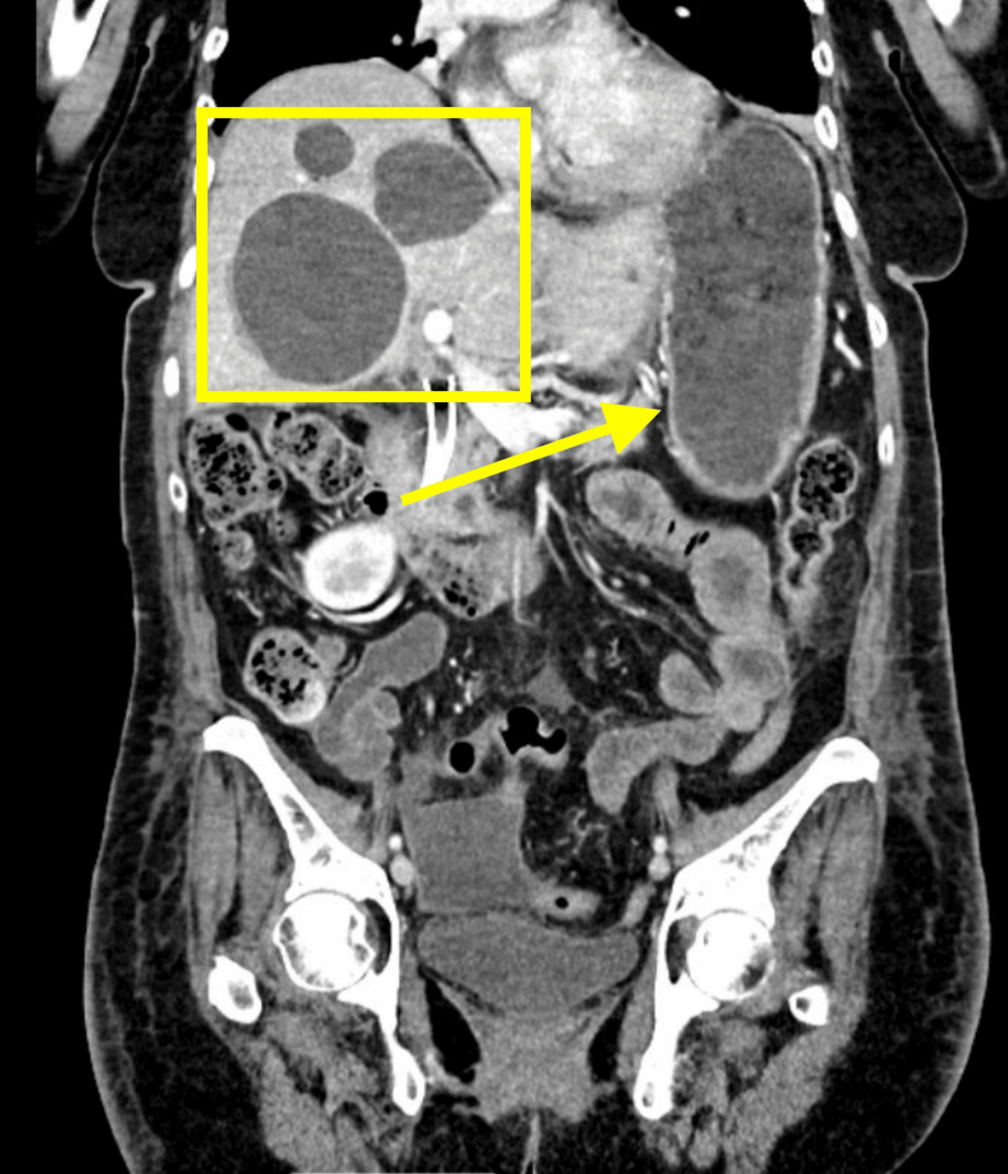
Multiple hepatic cysts indicated by a yellow square and splenic cyst indicated by a yellow arrow on CT scan CT: computed tomography
